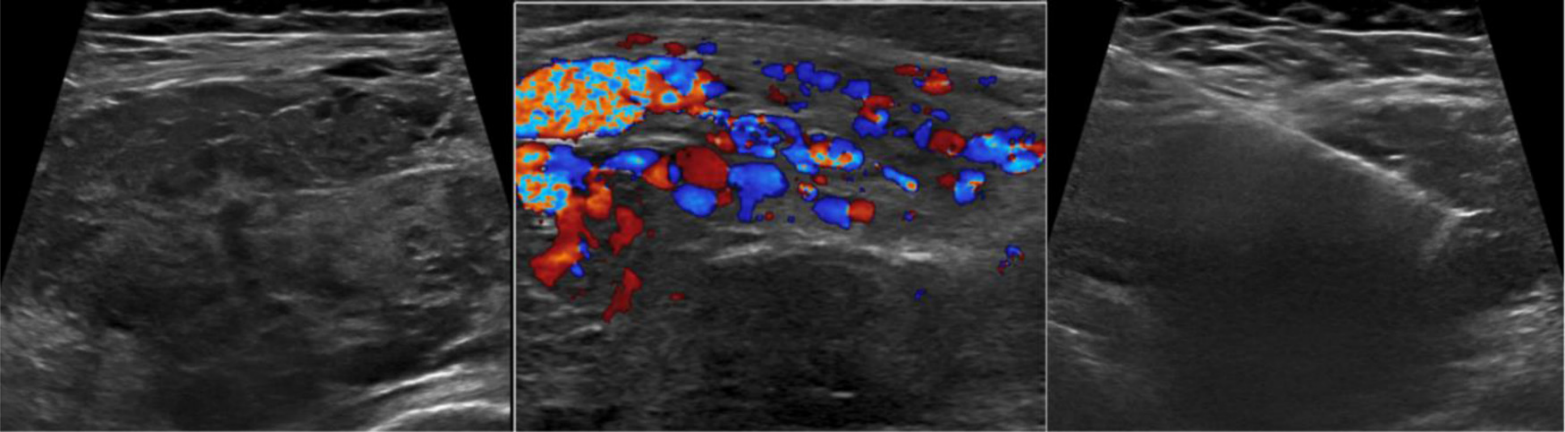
Ultrasound scan. (Left) There is a lobulated hypoechoic intramuscular mass at the right gluteal region. (Middle) The mass demonstrated pronounced vascularity within and around the lesion, associating with prominent vessels. (Right) Ultrasound guided biopsy of the right gluteal mass done.
